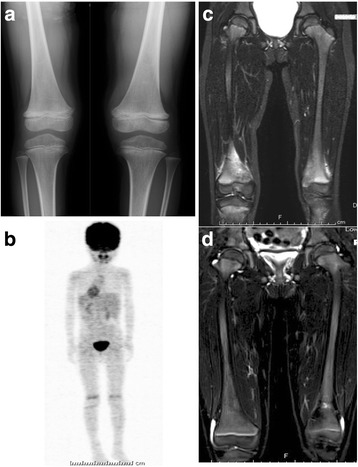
Representative case of a 5-year-old girl with CNO. The patient had a 3-month history of pain in both knees, and achieved clinical remission after the initial treatment. (a) Radiographic examination showed sclerosis in the distal metaphysis of both femurs. (b) FDG-PET image demonstrating increased multiple FDG uptakes in both distal femurs and right proximal tibia. (c) T2-STIR MRI scan at first presentation showing high signal in both distal femurs and right proximal tibia. (d) T2-STIR MRI scan at 6 months after the initiation of first-line treatment showing remission of high signals in both femurs and right tibia. CNO, chronic nonbacterial osteomyelitis; FDG-PET, fluorodeoxyglucose-positron emission tomography; T2-STIR MRI, T2-signal intensity in short tau inversion recovery magnetic resonance imaging
